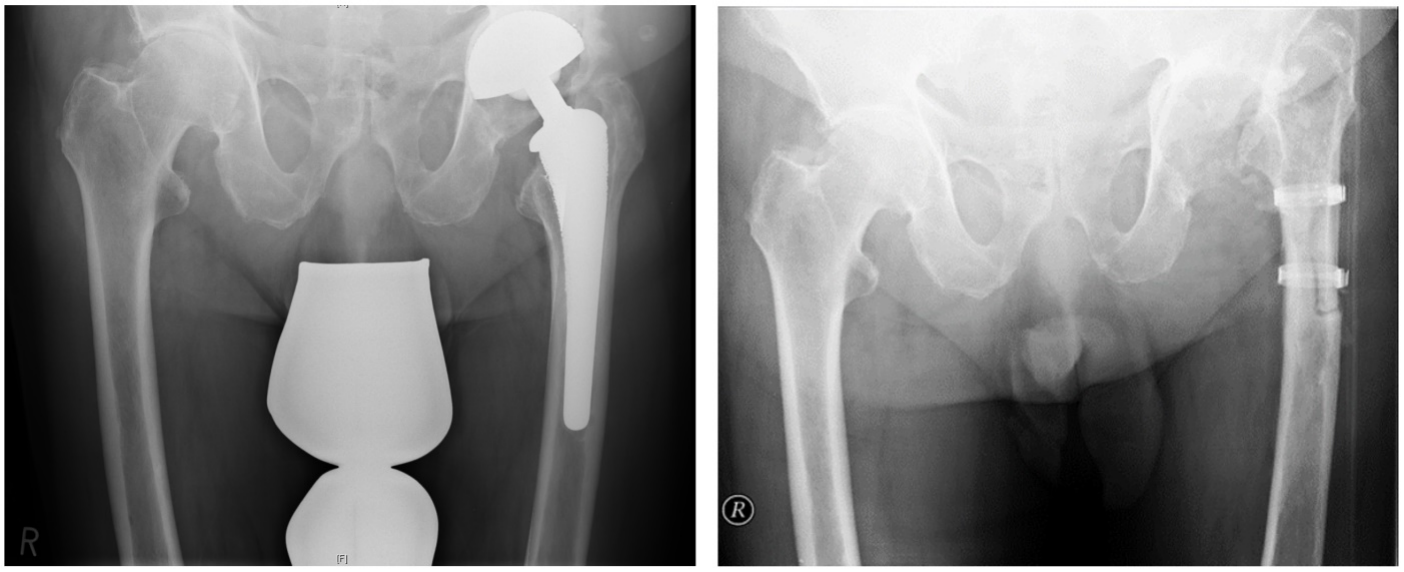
Pre- and postoperative AP pelvic radiograph of an infected THA. Explantation was performed by using an extended trochanteric osteotomy and re-fixation with two cerclages.
